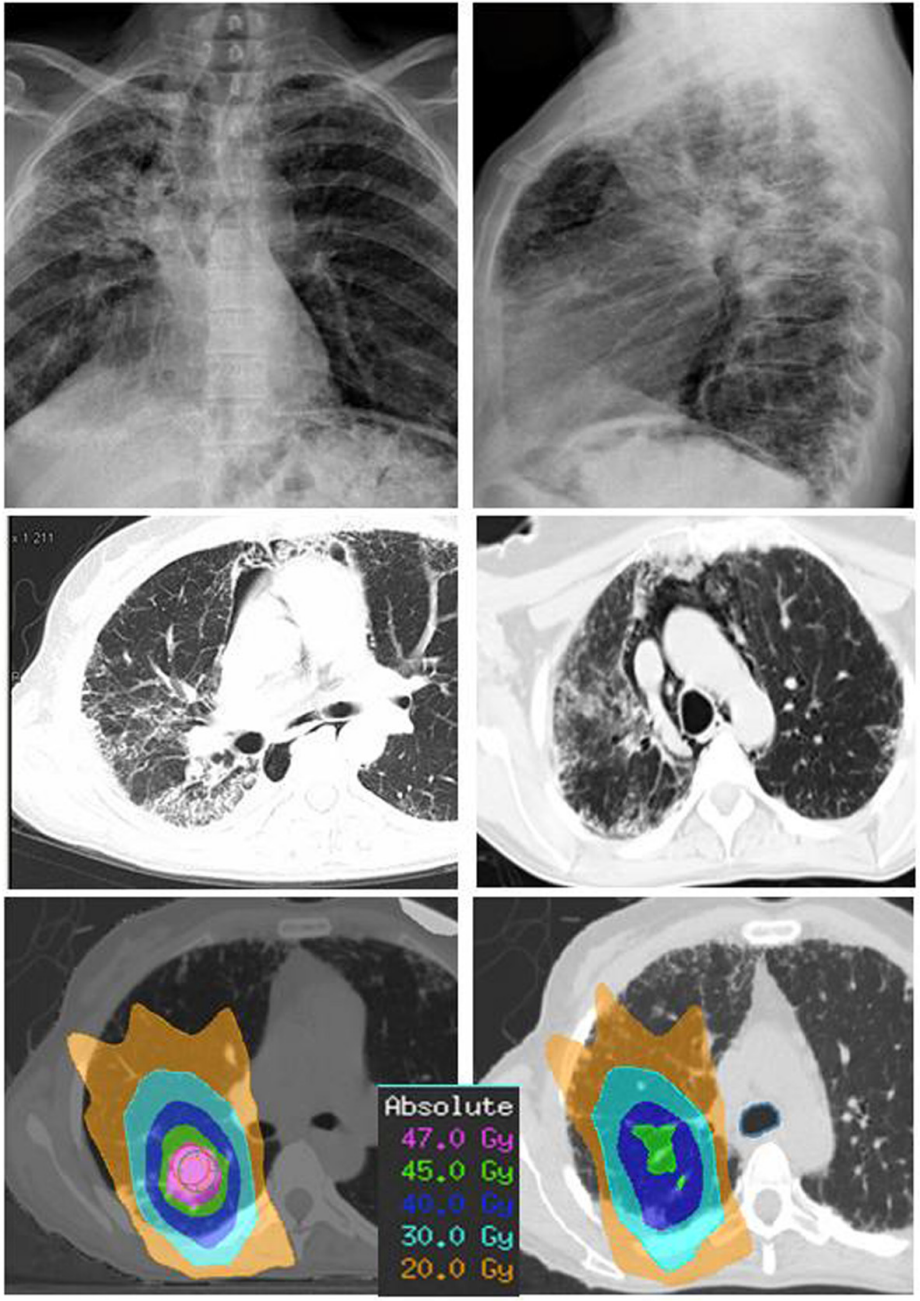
AP and lateral view of Chest X-ray show the presence of free air in the mediastinal cavity. Transverse Chest computed tomography (CT) after SBRT shows ground-glass opacities, bleeds confined to the right upper lung, and diffuse ground-glass attenuation, bleeds in the marginal areas, airspace consolidation, free air in the mediastinal cavity and fibrosis in the bilateral upper and lower lung fields. Transverse chest CT view of radiation dose distribution in the treatment course. Pink, green and dark blue, light blue and orange areas represent 47 Gy, 45 Gy, 40 Gy, 30 Gy and 20 Gy, respectively.
